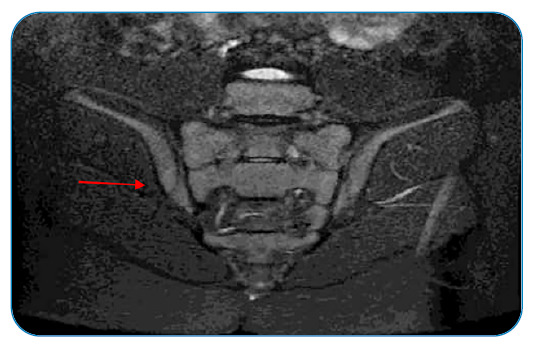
MRI showing resolution of the effusion.
